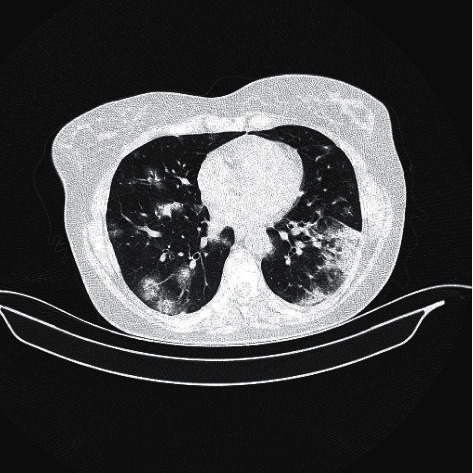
Axial CT image shows bronchocentric and nodular organizing pneumonia patterns and ground-glass opacifications.
